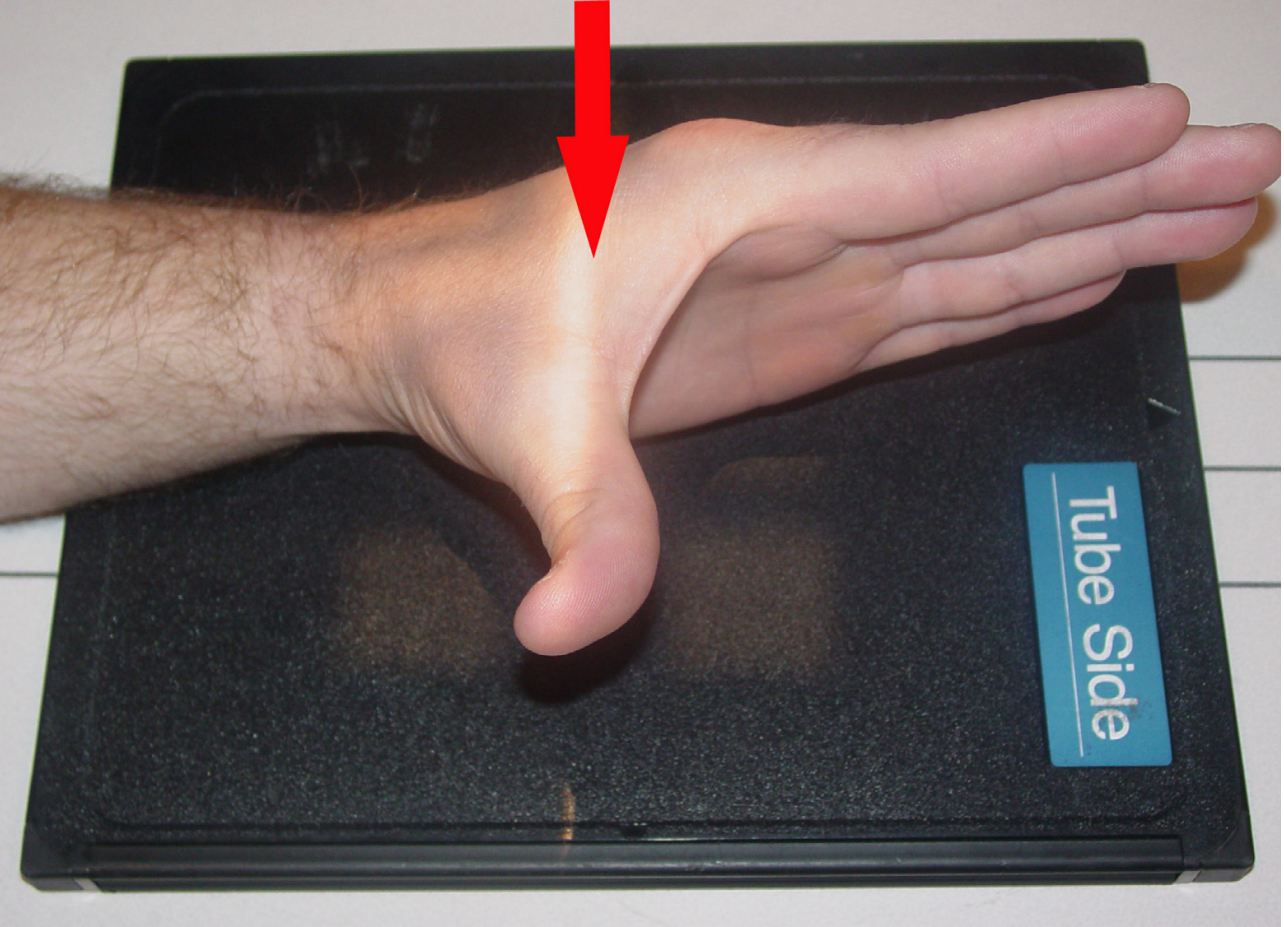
Radiographic position for radial-deviated, thumb-abducted view.
